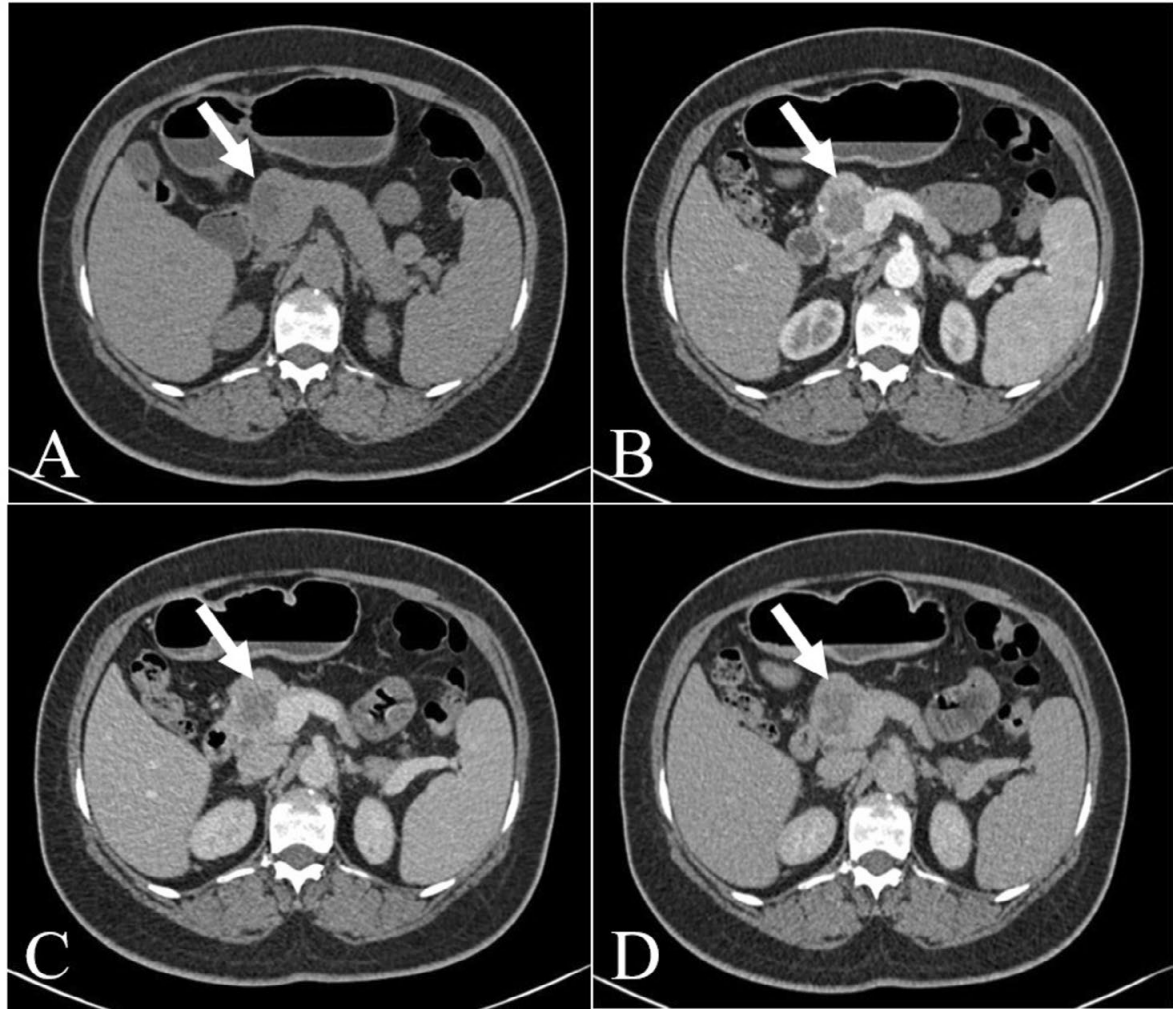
Non‐enhanced computed tomography (CT) revealed a round, well‐defined, and low‐density lesion in the head of the pancreas, measuring about 2.5 × 1.5 × 2.0 cm. Additionally, no significant calcification was observed (A). Enhancement scans showed internal segments of the lesion with mild to moderate enhancement and internal cystic components with no enhancement, which appear as a multilocular or honeycomb shape (B–D).
